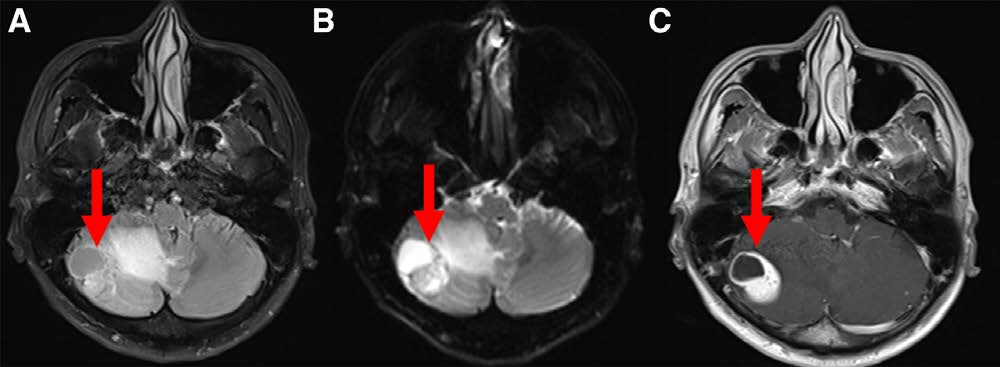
Brain MRI image of the patient. The T2WI/FLAIR (A), of the right cerebellar hemisphere mass (size, 3.2 × 2.4cm) is mixed with high signal intensity, accompanied by large edema signals at the edge, DWI (B) shows equal/low signal intensity, and the enhanced scan shows significant enhancement (C). MRI = magnetic resonance imaging.
